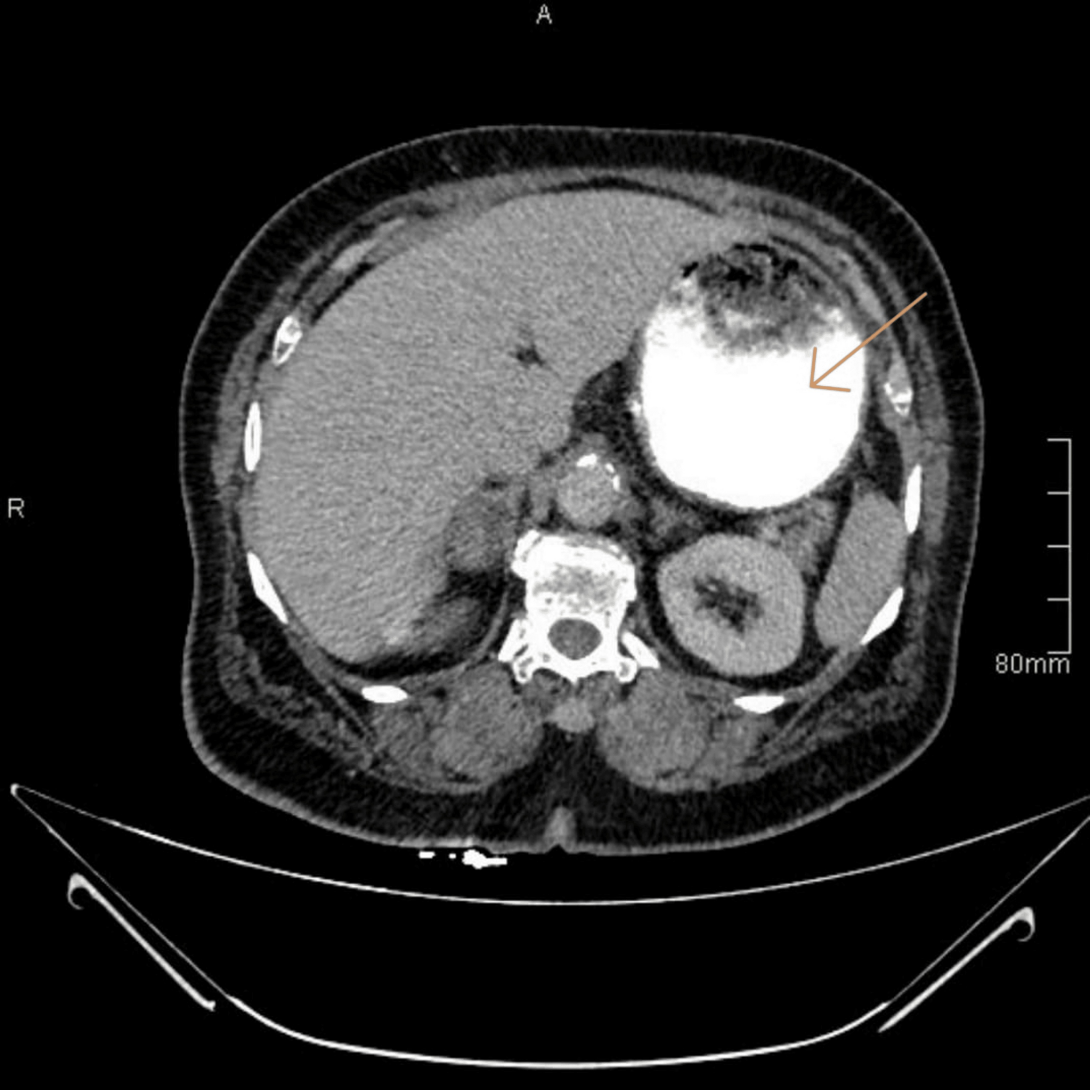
After surgery, abdominal CT, coronal section, with IV and oral contrast, shows no masses with stomach full of contrast; the oral contrast swallowed immediately before scanning
